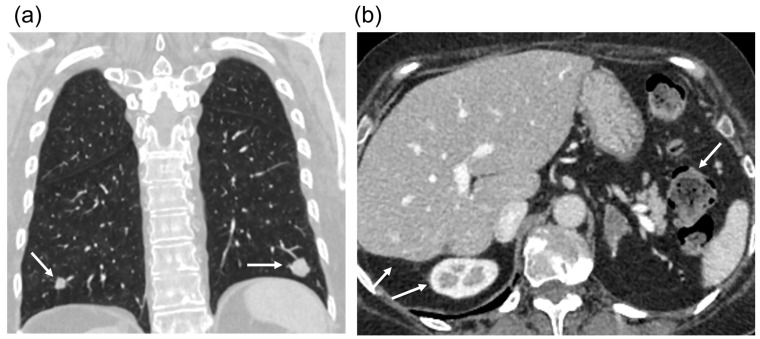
CT scans after 9 cycles of FOLFOX6 chemotherapy. (a) CT scan in coronal plane showing lung metastasis (arrows). (b) CT scan in venous phase showing the region of liver resection and the absence of the right adrenal gland (two arrows on the left); the left adrenal gland is preserved (arrow on the right).
